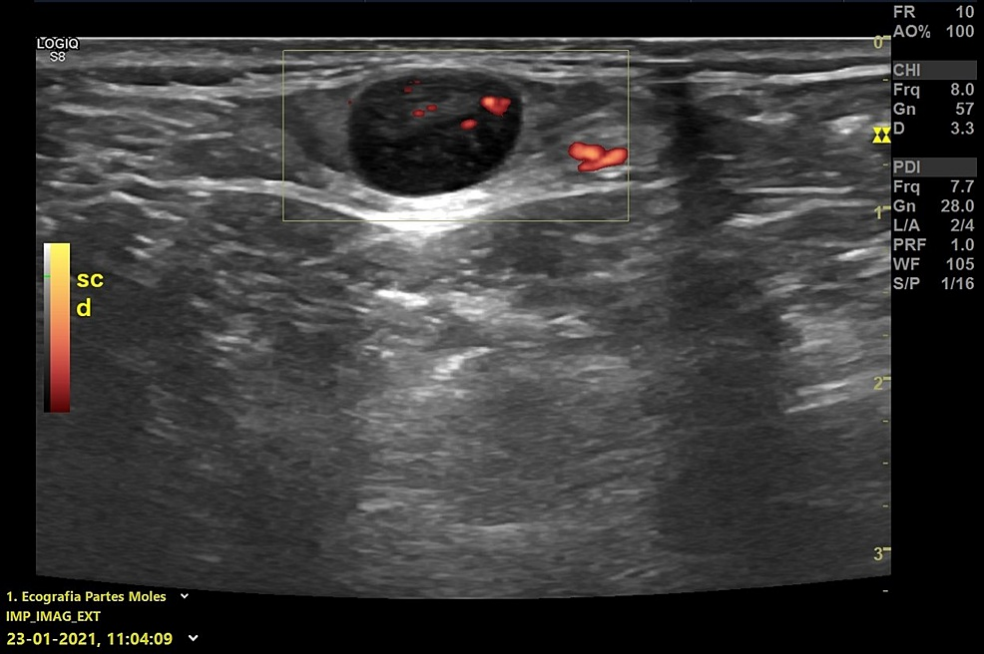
Ultrasonography - January 23, 2021 The image shows lymph node with an increase in echogenicity and in the sphericity index, without a defined hilum and 10.56 × 7.39 mm in size
